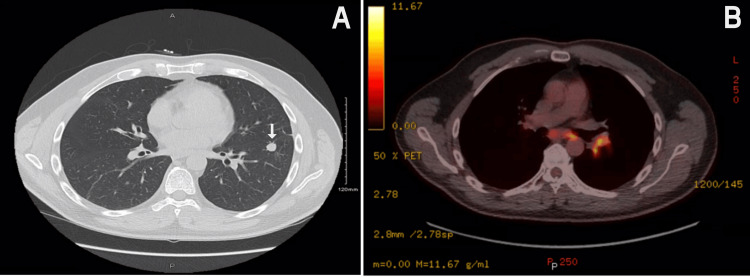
Axial CT scan of the chest without contrast demonstrating left lung mass 11.8 × 11.8 mm (A) and axial PET image demonstrating increased uptake in hilar lymph nodes (B)
